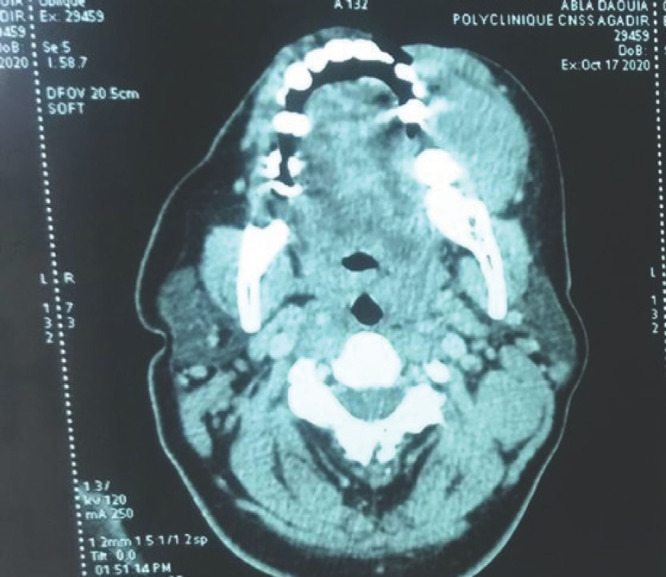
Tomodensitométrie cérébro-faciale montrant un processus tumoral gingivale gauche au contact du corps mandibulaire Cerebrofacial computed tomography showing a left gingival tumor process in contact with the mandibular body
